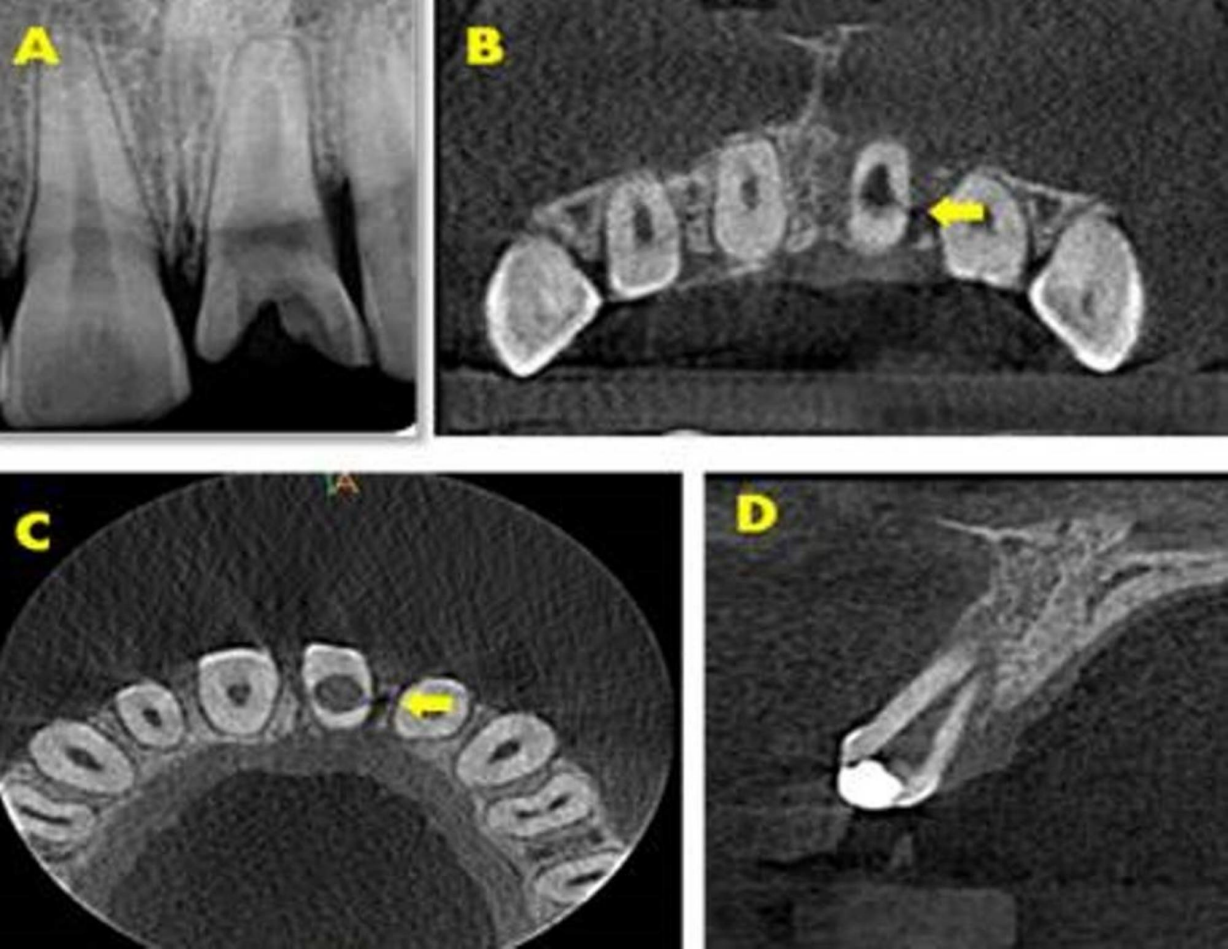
Preoperative assessment for inflammatory perforating internal root resorption. (A) Preoperative radiograph with enlargement of root canal space suggesting internal root resorption. (B) Axial section of CBCT showing communication with external root surface. (C) Axial section of CBCT with internal root resorption and perforating with external root in the coronal third. (D) Sagittal section showing enlargement of root canal suggesting internal root resorption. CBCT: Cone beam computed tomography
